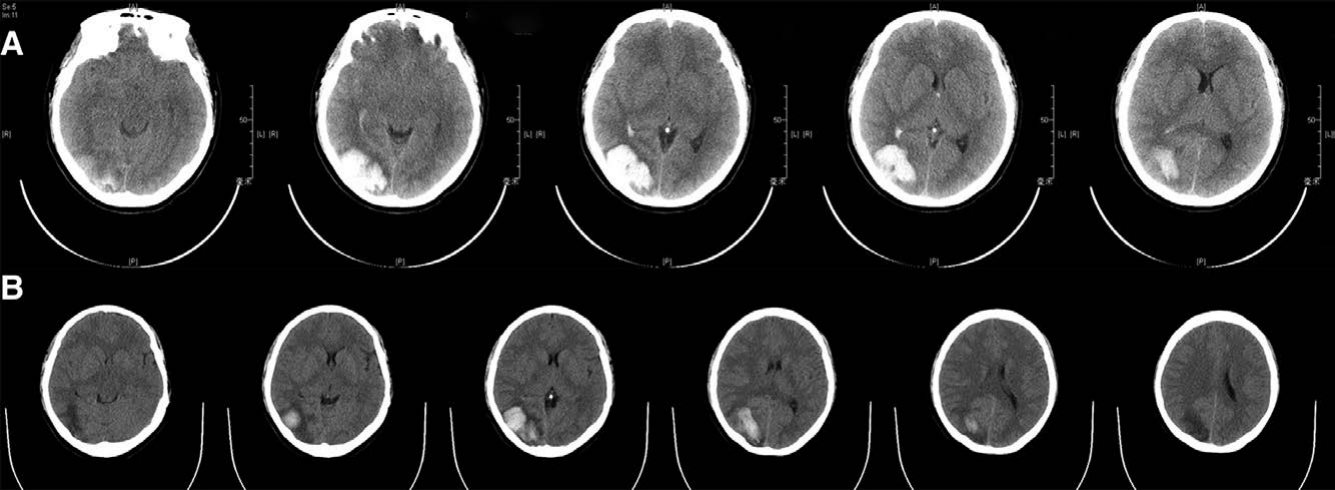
(A) The CT results of the brain within 24 hours after the operation. (B) The CT results of the brain indicating the patient’s treatment status at the time of discharge. CT = computed tomography.
